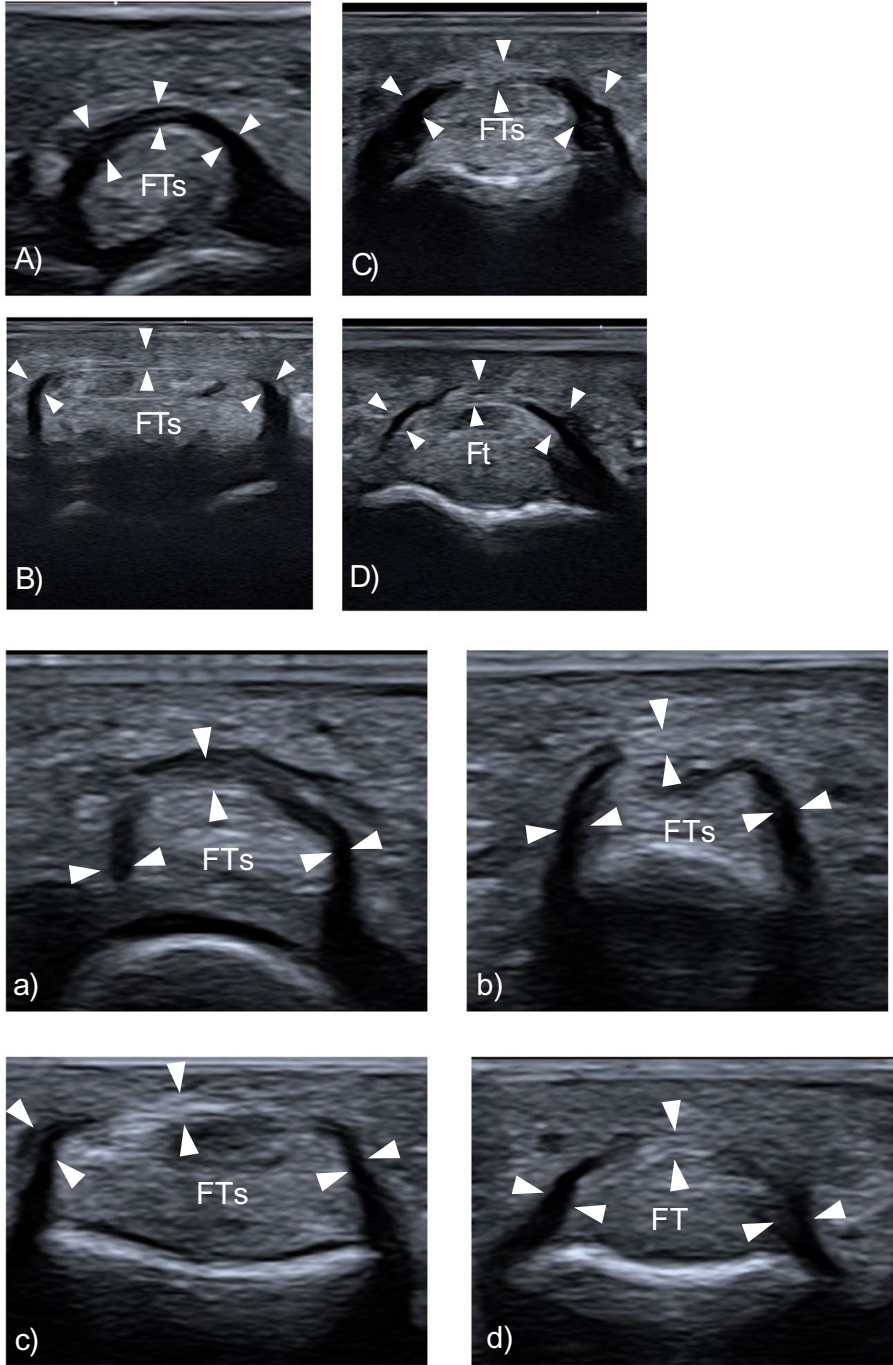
Normal ultrasound appearances of annular pulleys in healthy individuals. Transverse US images of the annular pulleys of the II toe obtained with a 22–8 MHz hockey-stick probe (A) and an 18–5 MHz probe (a). A-a A1 pulley (arrowheads) overlying the flexor tendons (FTs) at the level of the metatarsophalangeal joint. B-b A2 pulley (arrow-heads) overlying the flexor tendons (FTs) at the level of the proximal phalanx. C–c A3 pul- ley (arrowheads) overlying the flexor tendons (FTs) at the level of the proximal interphalangeal joint. D-d A4 pulley overlying the flexor digitorum longus tendon (Ft) at the level of the distal interphalangeal joint. FTs, flexor tendons; FT, flexor tendon
